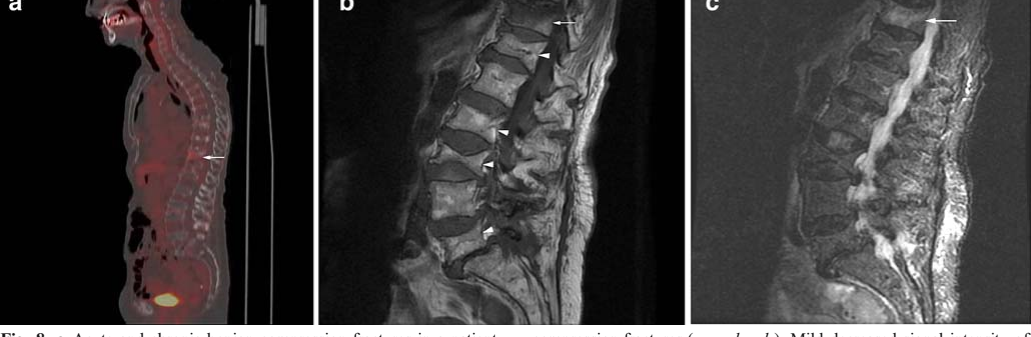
a Acute and chronic benign compression fractures in a patient without history of malignancy. Sagittal fused FDG-PET/CT image demonstrates multiple compression fractures throughout the thoracic and lumbar spine with mild increased radiotracer uptake at T11 (SUV = 2.1;* arrow*). b Sagittal T1-weighted MRI demonstrates multiple chronic compression fractures (*arrowheads*). Mild decreased signal intensity of the T11 compression fracture suggests edema (*arrow*). c Sagittal STIR image demonstrates mild edema in the T11 compression fracture suggestive of an acute fracture (*arrow*). There is no evidence of marrow edema in the remaining compression fractures
